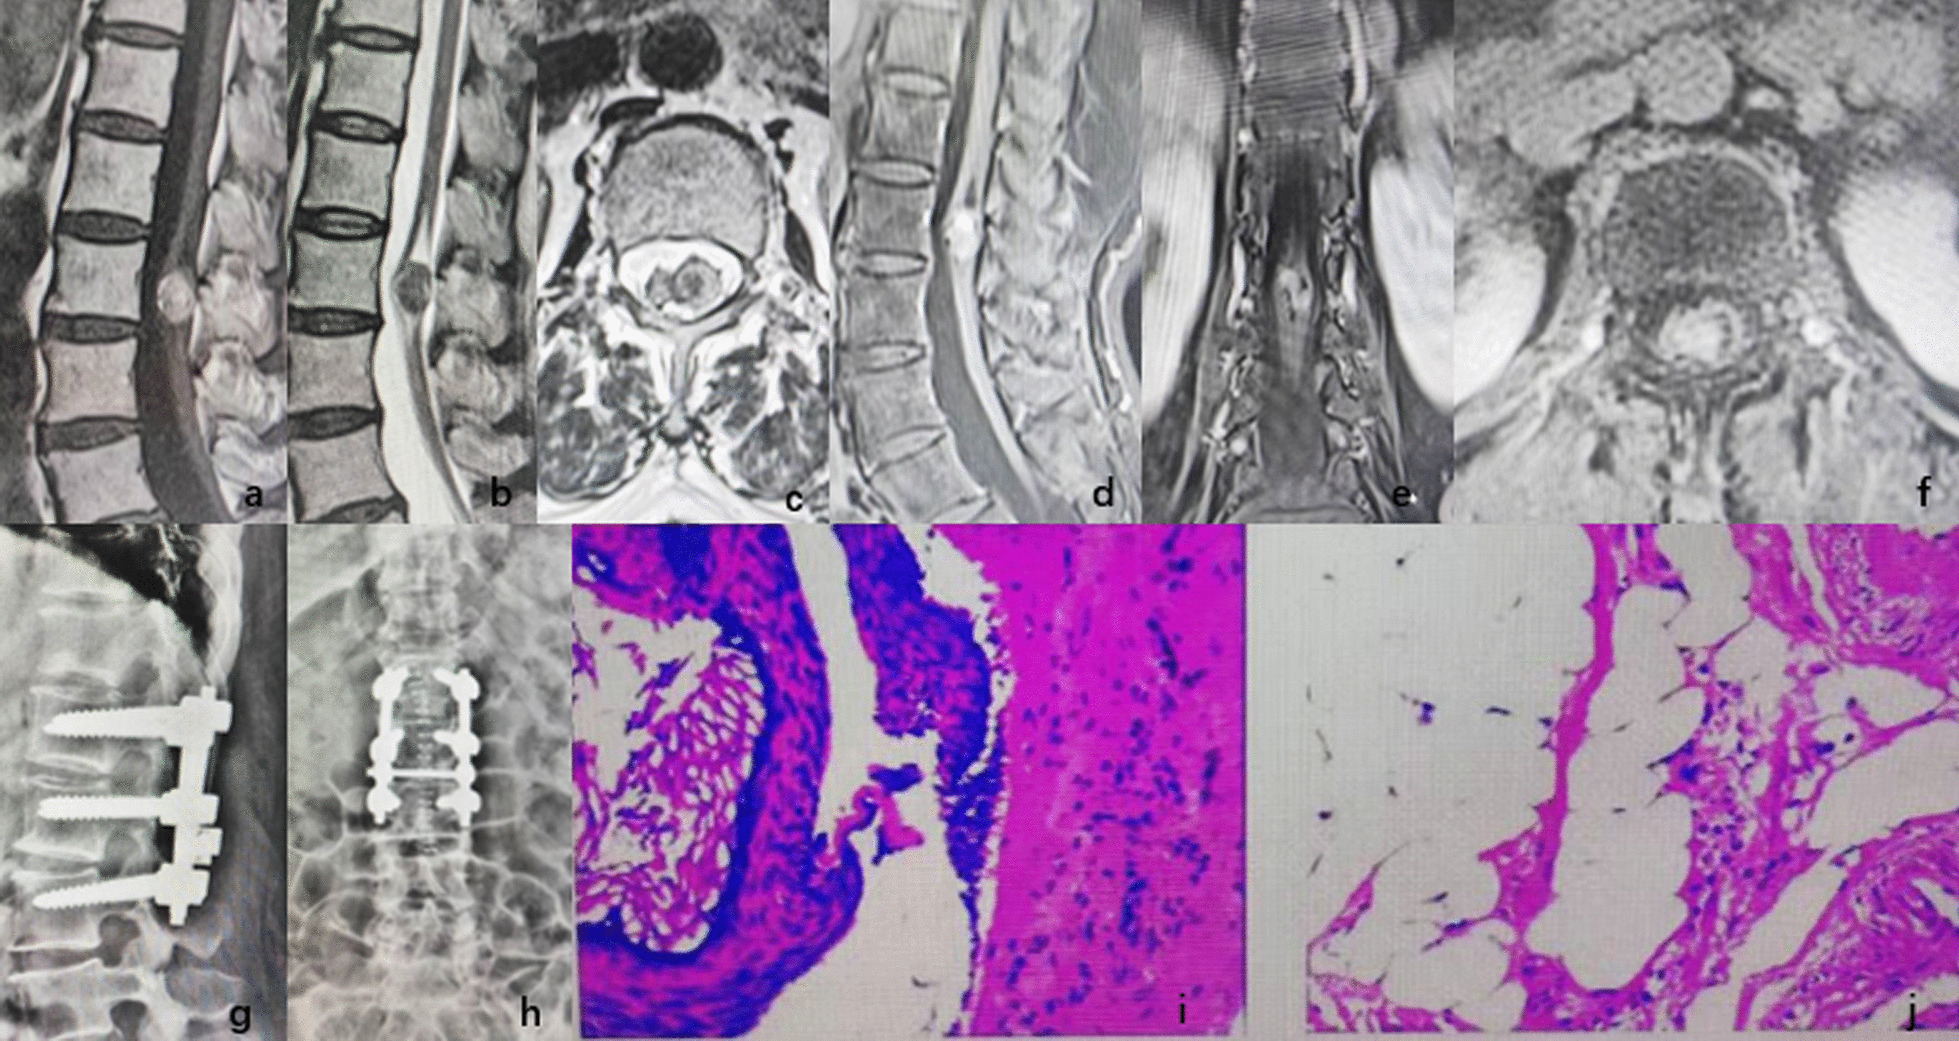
a T1WI image of preoperative MRI, b T2WI image of preoperative MRI, c cross-sectional image of preoperative MRI, d sagittal position of preoperative enhanced MRI, e coronal position of preoperative enhanced MRI, f cross-sectional image of preoperative enhanced MRI, g and h pedicle screw fixation after tumour resection, i and j: mature cystic teratoma shown by pathology
